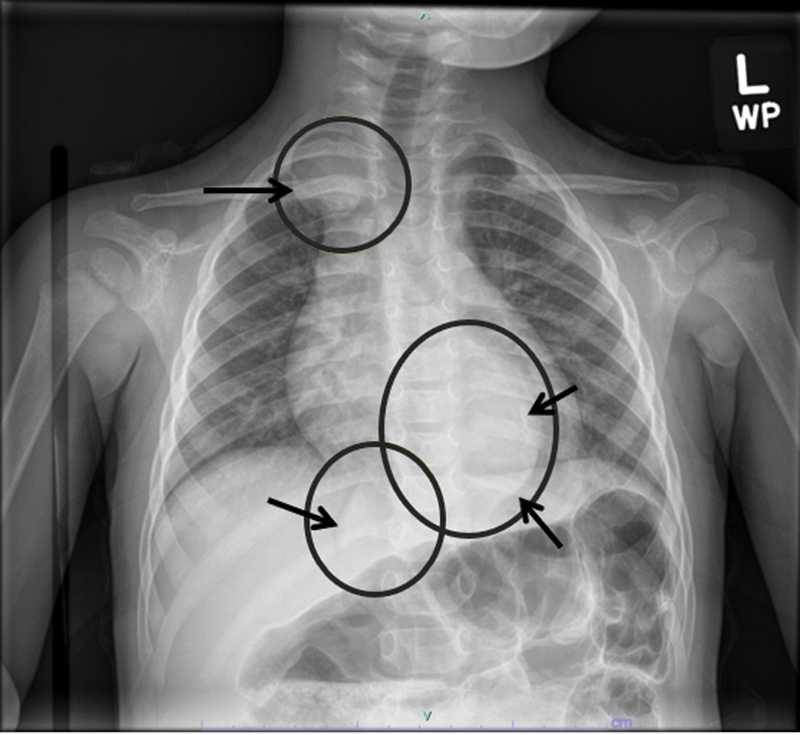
A dedicated chest X-ray showing three masses annotated with circles and arrows.
